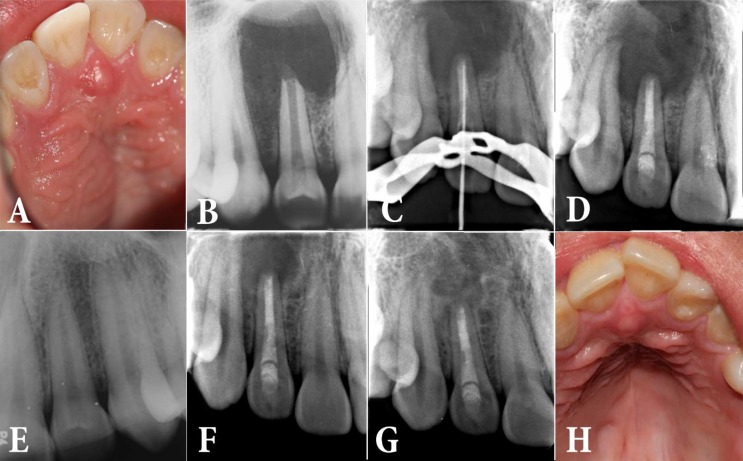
A)* Preoperative periapical radiography of the right maxillary lateral incisor; *B)* Palatal swelling; *C)* Periapical radiography following periapical surgery; *D)* Penetration of an endodontic instrument into the root-end filling material; *E)* The radiography of left counterpart; *F)* Filling the root canal space and the unset part of Angelus MTA with CEM cement; *G)* Healed periapical lesion 27 months following the treatment; *H)* Palatal swelling has healed
